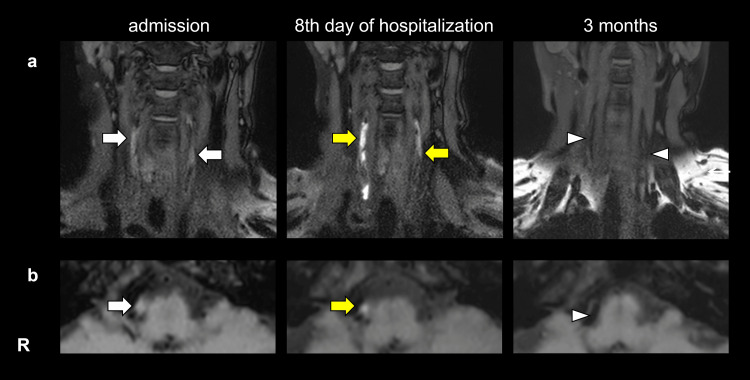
3D T1-weighted head and neck black-blood imaging (BBI) a. Changes in 3D T1-weighted imaging in the V1 segment of both vertebral arteries. On admission, 3D T1-weighted imaging revealed slight intramural hematomas in the bilateral V1 segments in both vertebral arteries (white arrows). Follow-up imaging on the eighth day of hospitalization revealed an increase in the signal intensity of the intramural hematomas (yellow arrows). Three months later, the intramural hematomas had completely resolved (white triangle). b. Changes in the BBI in the V4 segment of the right vertebral artery. On admission, BBI revealed slight intramural hematomas in the right vertebral artery (white arrow). Follow-up imaging on the eighth day of hospitalization revealed an increase in the signal intensity of the intramural hematomas (yellow arrow). Three months later, the intramural hematomas had completely resolved (white triangle).
